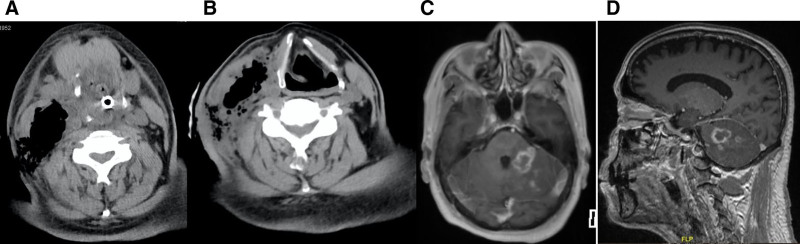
(A) The patient’s cervical CT on March 25 revealed multiple gas-dense shadows in the right cervical sternocleidomastoid muscle and subcutaneous soft tissues, with surrounding soft tissue swelling and blurring. (B) A repeat CT scan of the neck on April 3 revealed a slight improvement in the size of the neck mass and the severity of the soft tissue infection in comparison to the previous scan. (C) and (D) A head enhancement MRI conducted on April 20 revealed the presence of irregular signal shadows on the left side of the head, particularly in the left pontine arm and the left cerebellar hemisphere.
